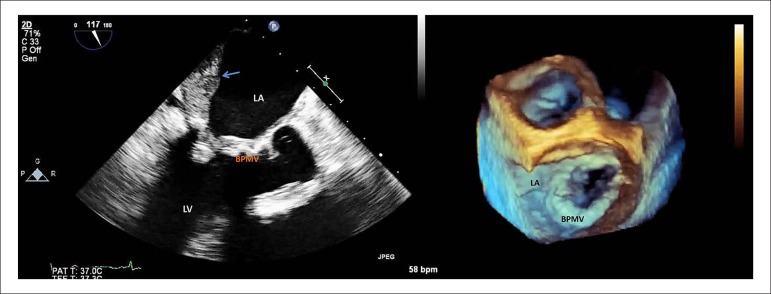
Transoesophageal echocardiogram long axis view and 3D zoom on face
